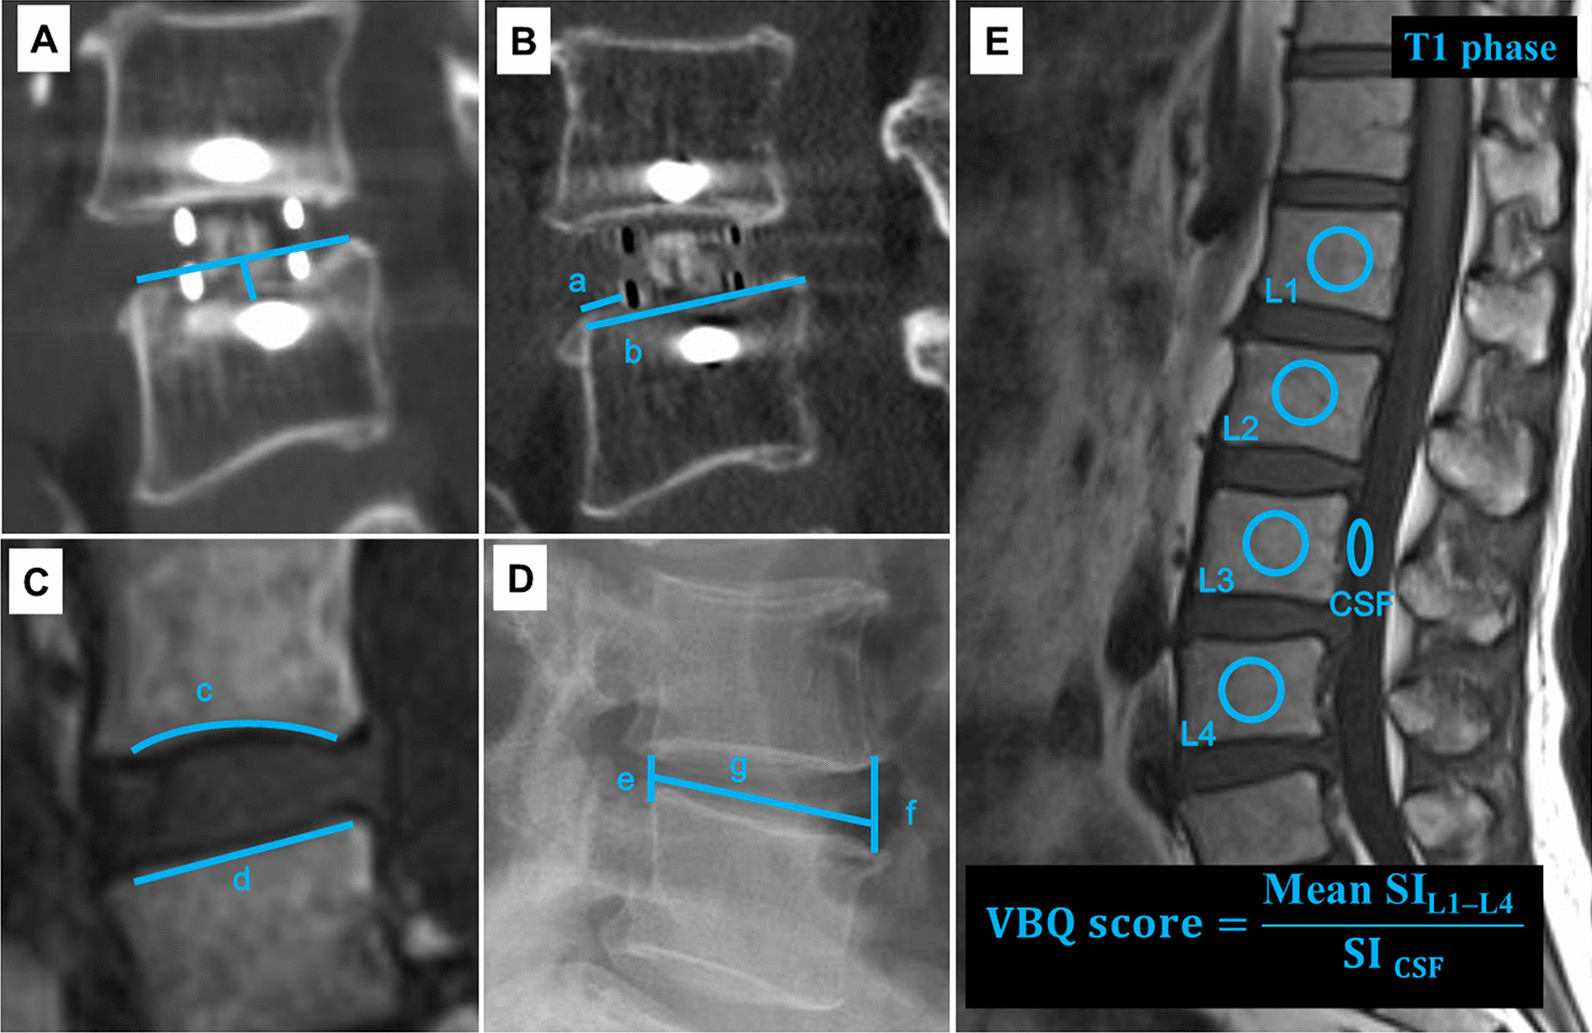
A Cage subsidence is indicated as ≥ 2 mm sinking of the cage into the superior endplate. B The cage position is calculated as $$\frac{a}{b} \times 100\%$$ (a, the distance between the anterior metal marker and the leading edge of the caudal endplate; b, he length of caudal endplate). C Classification of the disk space morphology on MRI scan (c, concave; d, fat). D The disk height is calculated as $$\frac{e + f}{g} \times 100\%$$ on a lumbar X-ray (lateral view) (e, anterior disk height; f, posterior disk height; g, sagittal disk width). E Representative image of the ROI used to calculate the VBQ score
